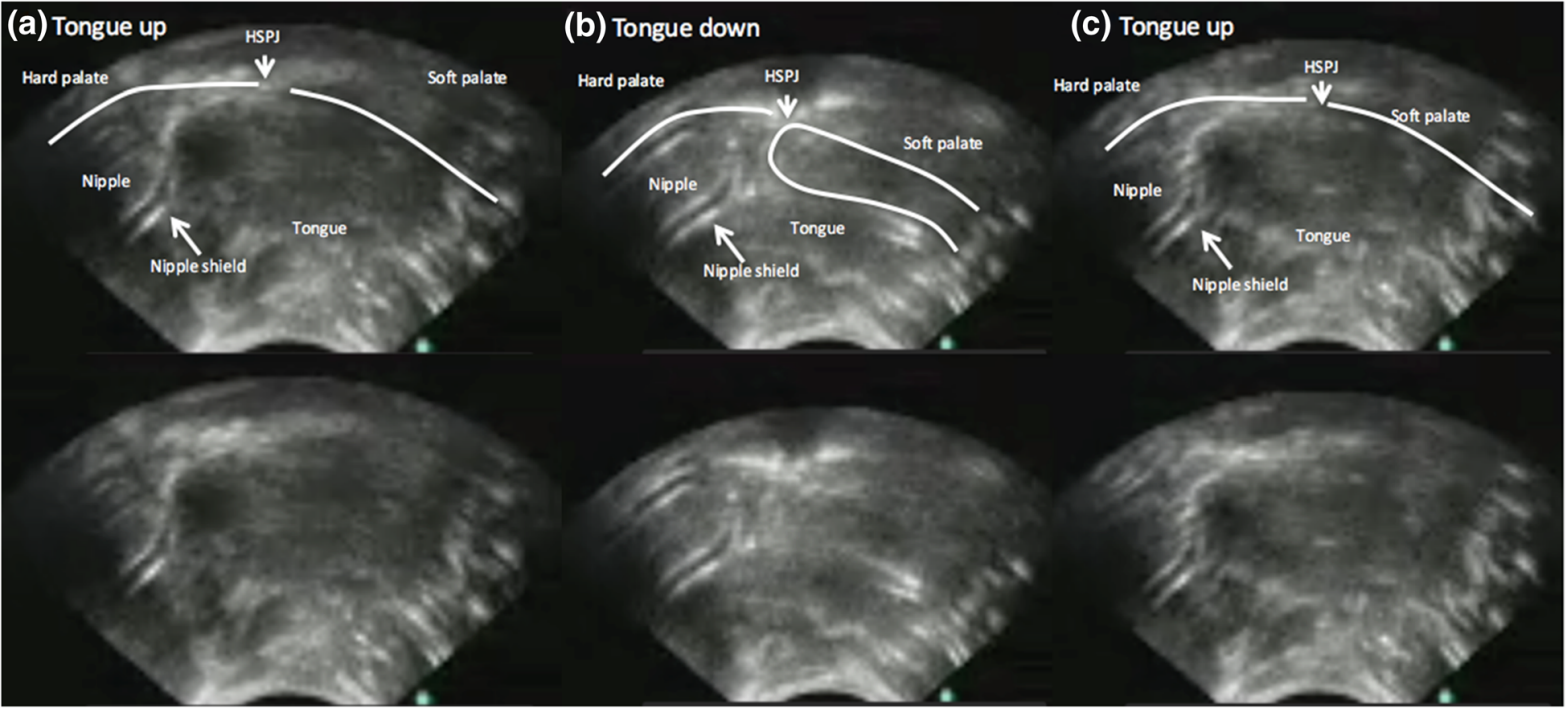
Ultrasound images of one cycle of a preterm breastfeeding infant feeding with a nipple shield (a) tongue up corresponds with baseline vacuum (b) when the tongue is lowered to the lowest point peak vacuum is applied to the breast and milk flows (c) tongue returns to the soft palate and milk is removed from the oral cavity
